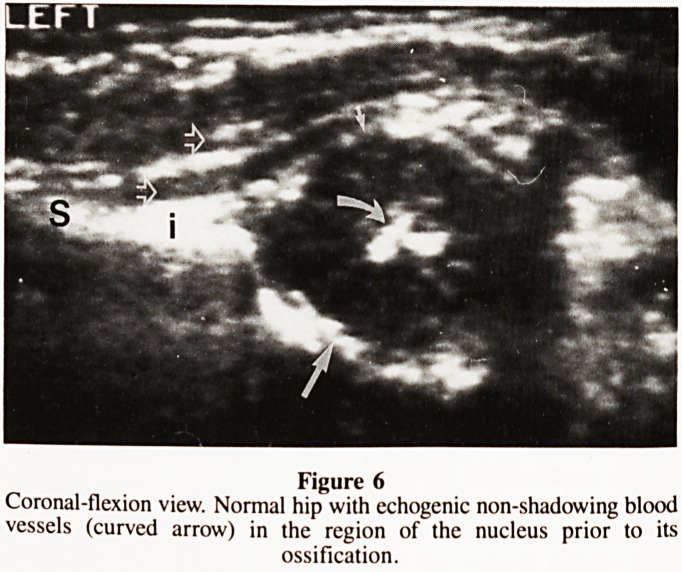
# Ultrasound of the Infant Hip
